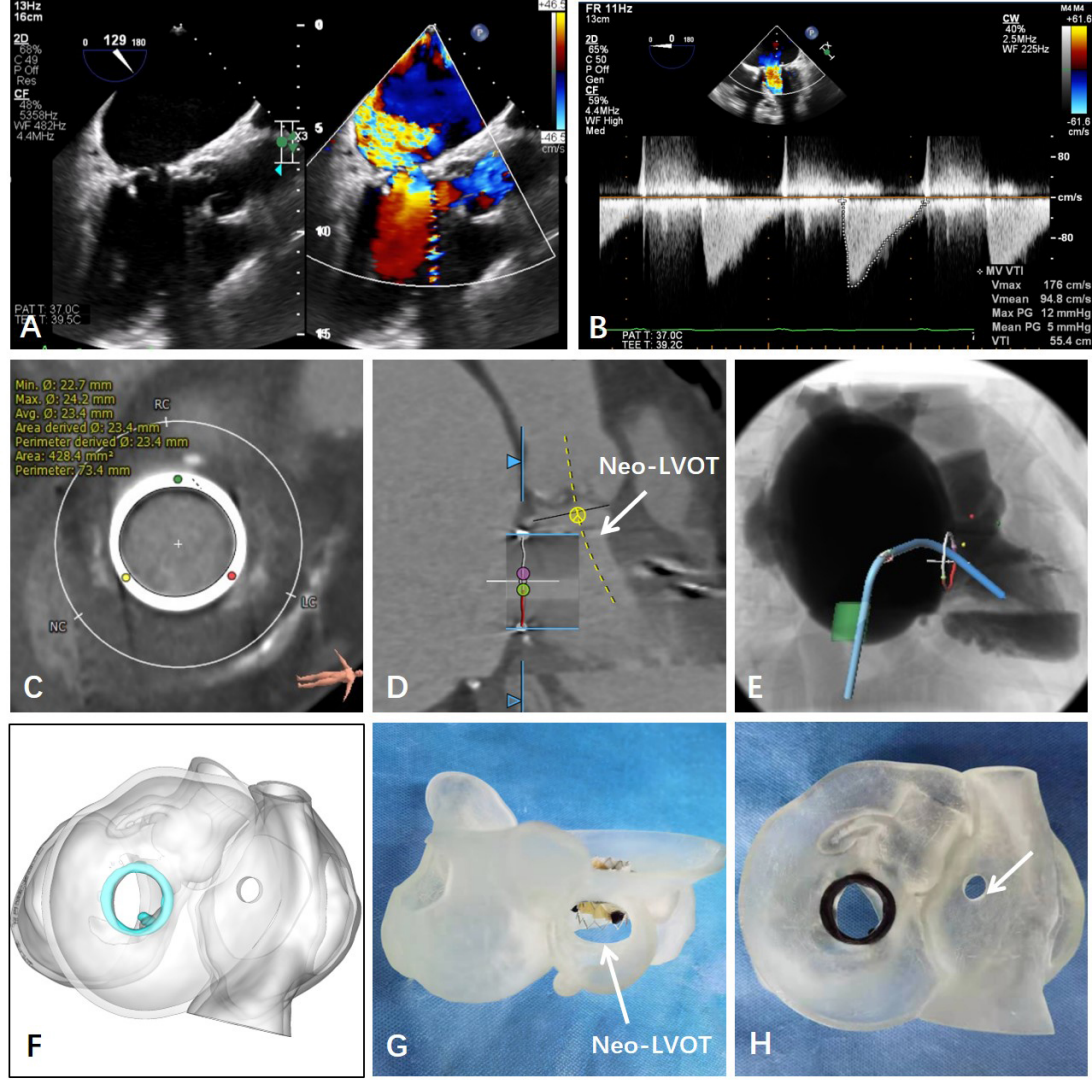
Preoperative measurements based on TEE, CT and 3D printing. (A)
